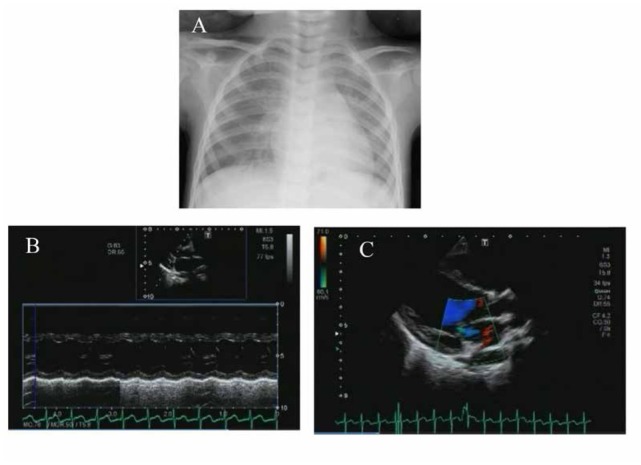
Image examinations on day 8 of illness A: Chest X-ray The cardio-thoracic ratio was 0.53 and congestion was observed in the hilum of both lungs. B and C: Echocardiograms The left ventricular ejection function was 39%, percent fractional shortening was 20.9%, and the left ventricular end-diastolic dimension was 33.9 mm (104% larger than in a normal case).
